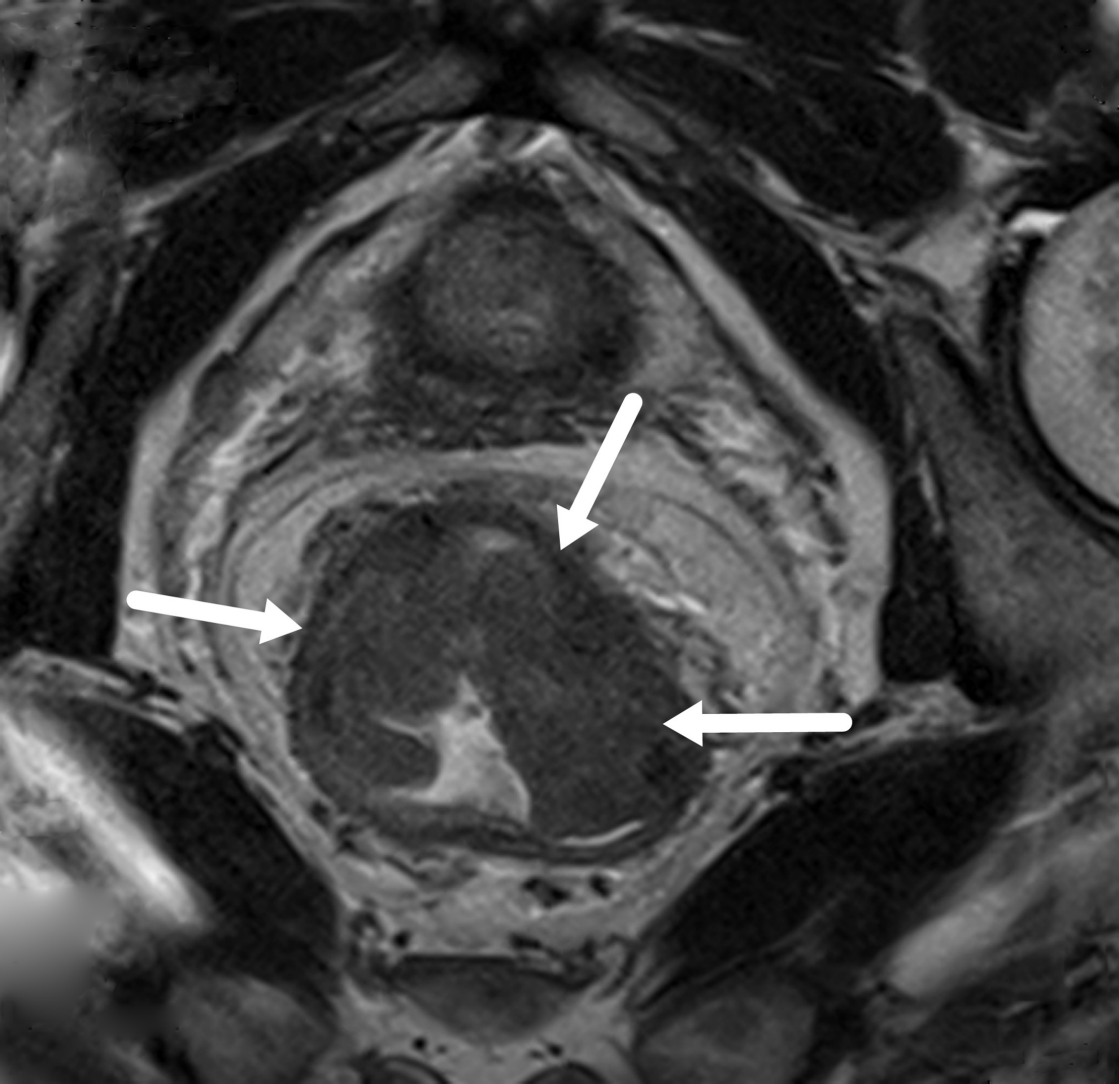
An MR image of a rectal carcinoma. A thickened rectum wall shows slightly higher signals on the T2WI map.
